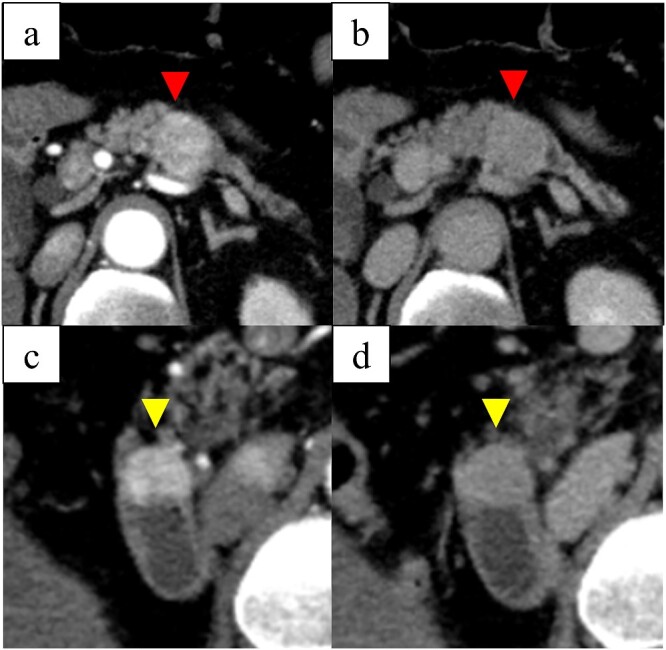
Computed tomography images: (a) arterial-phase axial computed tomography shows a hypervascular tumor in the pancreatic body, (b) the pancreatic tumor was washed out in the venous phase, (c) arterial-phase axial computed tomography shows a hypervascular tumor in the descending duodenum, and (d) the duodenal tumor was washed out in the venous phase.
